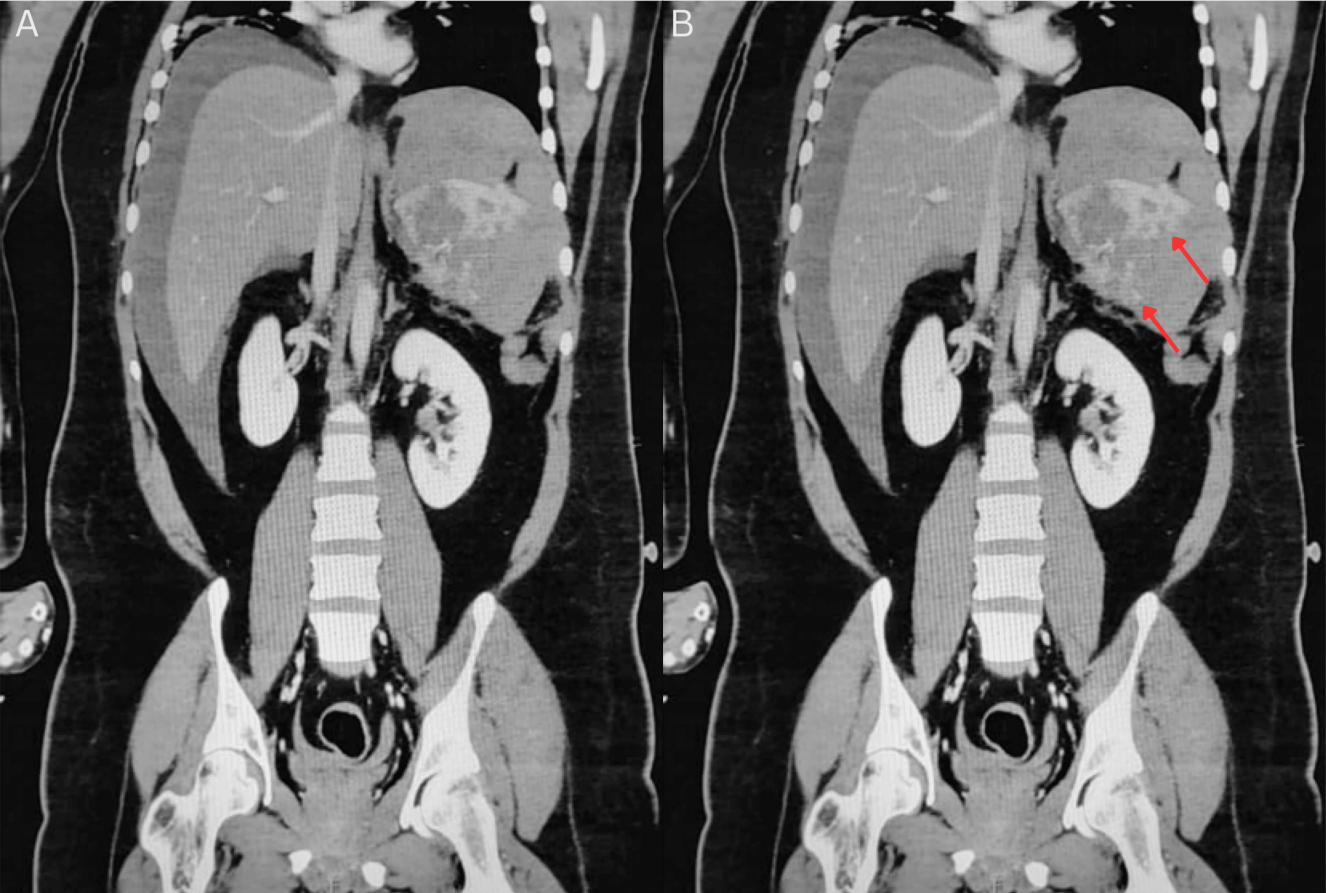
Contrast-enhanced coronal venous-phase CT of abdomen demonstrating splenic rupture Pre-operative coronal venous-phase contrast-enhanced CT suggestive of splenic parenchyma with focal areas of contrast extravasation (red arrows). (A) shows the unannotated scan and (B) shows the annotated scan.
